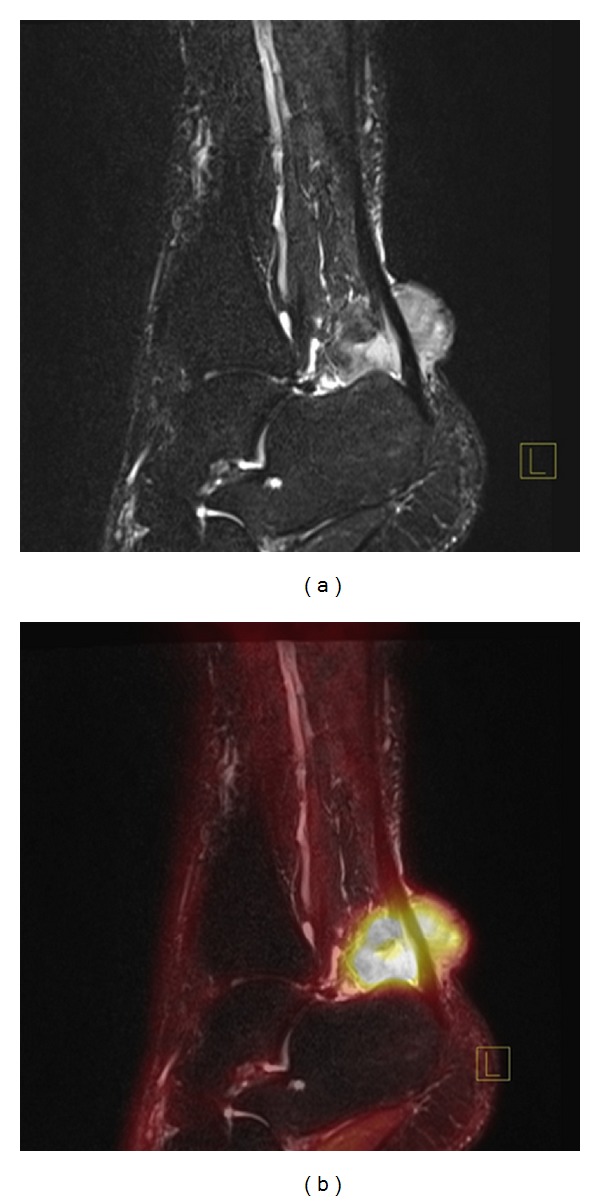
T1-weighted postgadolinium MRI shows a sagittal section of a clear cell sarcoma surrounding the Achilles tendon (Case 1), and tumor ingrowth to the calcaneus cannot be excluded (a). Fused FDG-PET and MRI show no tumor involvement of the bone (b).
